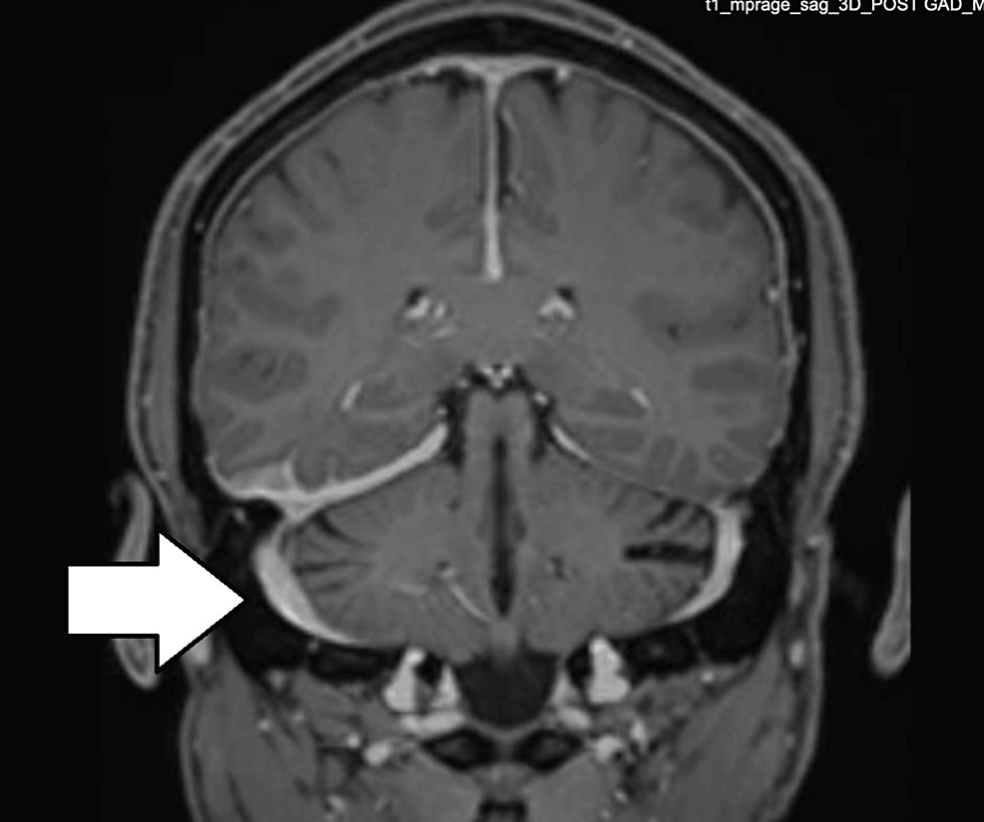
MRI of the brain: T1 sagittal contrast, enhanced view, showing contrast enhancement of the meninges, mainly in the right tentorium cerebellum
